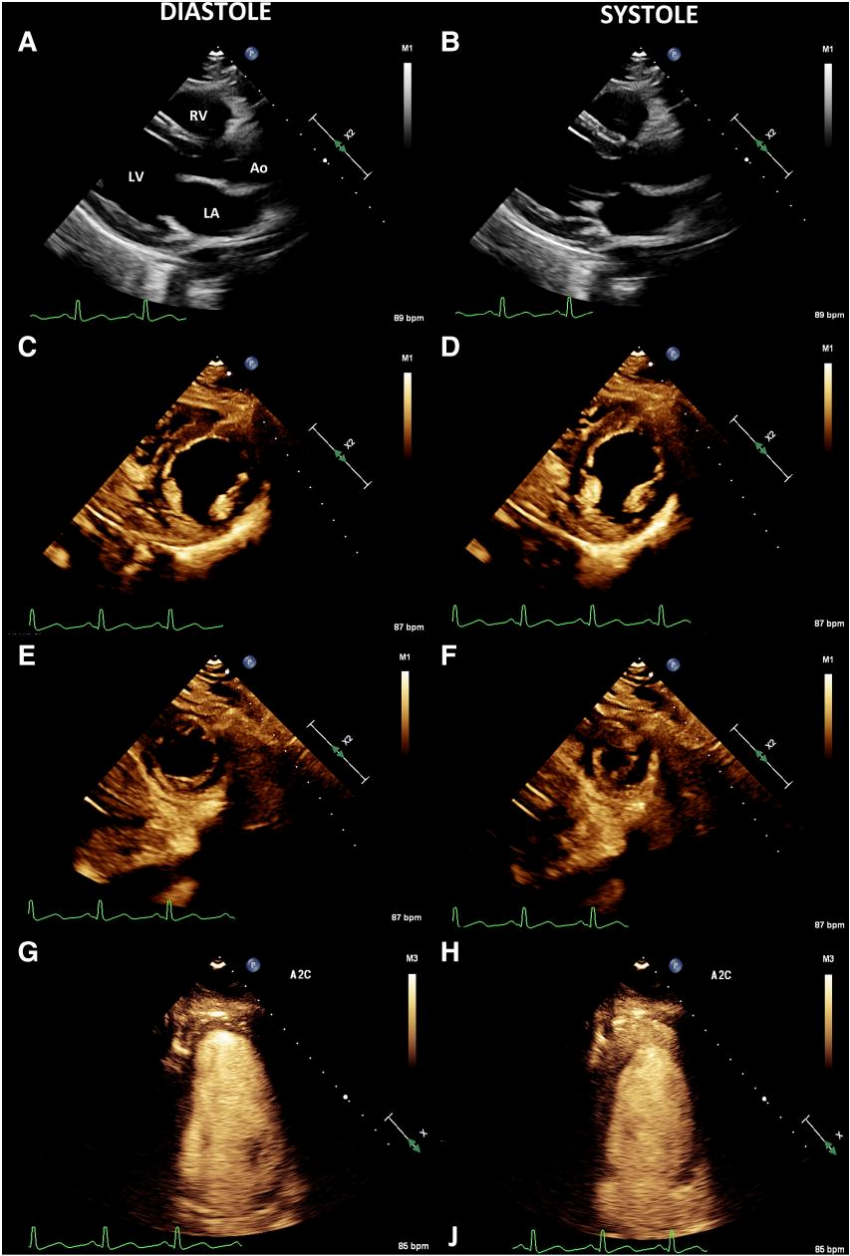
Transthoracic echocardiogram images (*A–H*) showing a reverse takotsubo-like cardiomyopathy pattern with preserved function of the left ventricular apex (*F*) and hypokinesis of the basal and septal segments (*B* and *D*).
